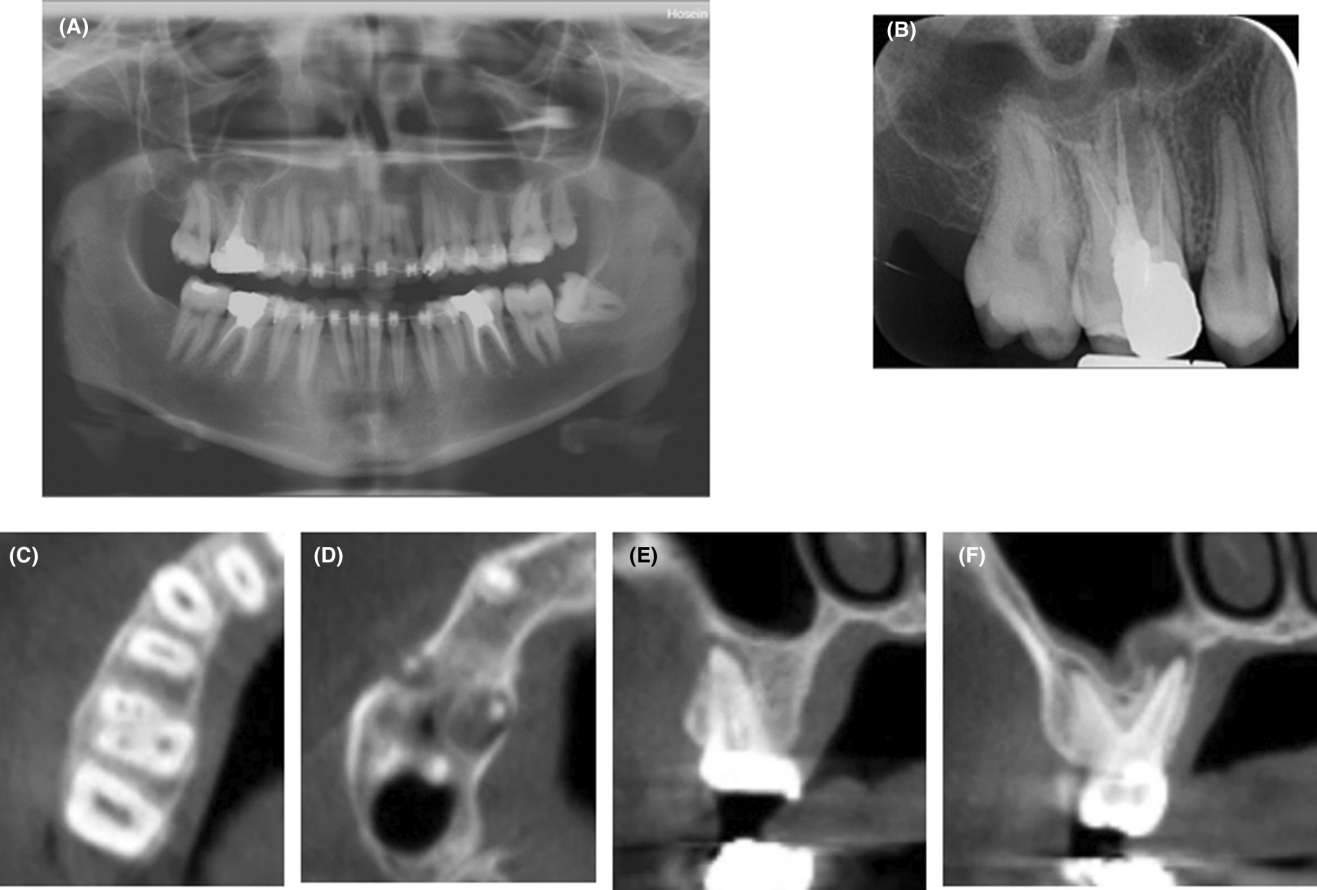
Panoramic view (A), diagnostic periapical radiograph (B), CBCT axial view of right maxillary first molar after the first session of retreatment (C), CBCT axial view showing periapical lesions related to the mesiobuccal and palatal roots; buccal cortex associated with mesiobuccal root apex is perforated (D), CBCT coronal view of mesiobuccal root associated with buccal cortex perforation (E), and CBCT coronal view of palatal and distobuccal roots, Note the mucosal thickening in the right maxillary sinus (F).
